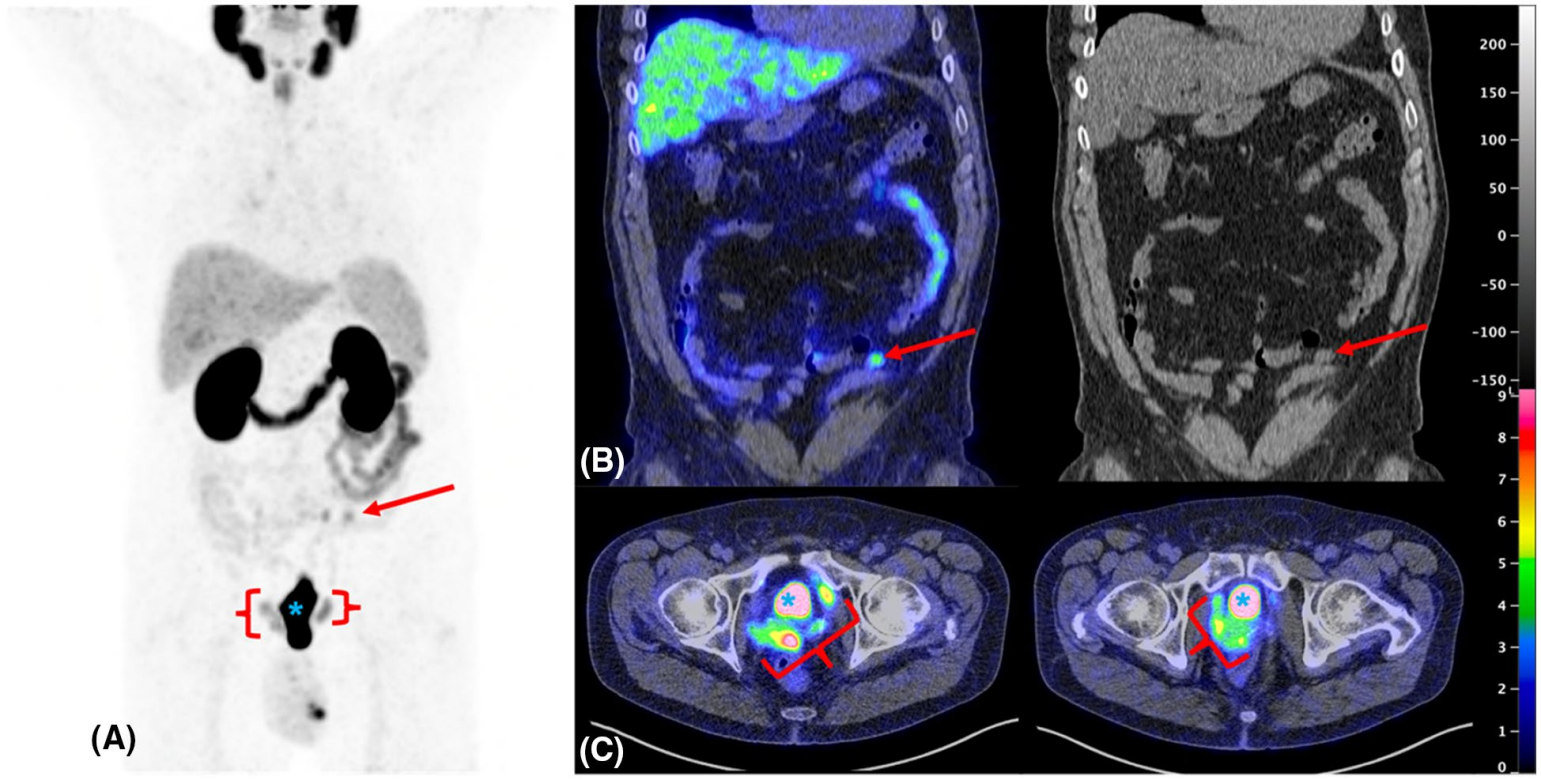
68Gallium PSMA‐PET of pelvic and abdominal disease 6 months after robot‐assisted radical prostatectomy (RARP). A clear mesenteric/peritoneal metastasis is visible (panel A & B, red arrow), alongside bulky pelvic disease (panel C, red bracket)
